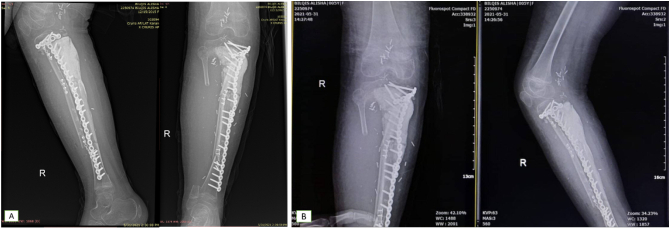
Post-operative X-ray of the: A) Cruris; B) knee.
